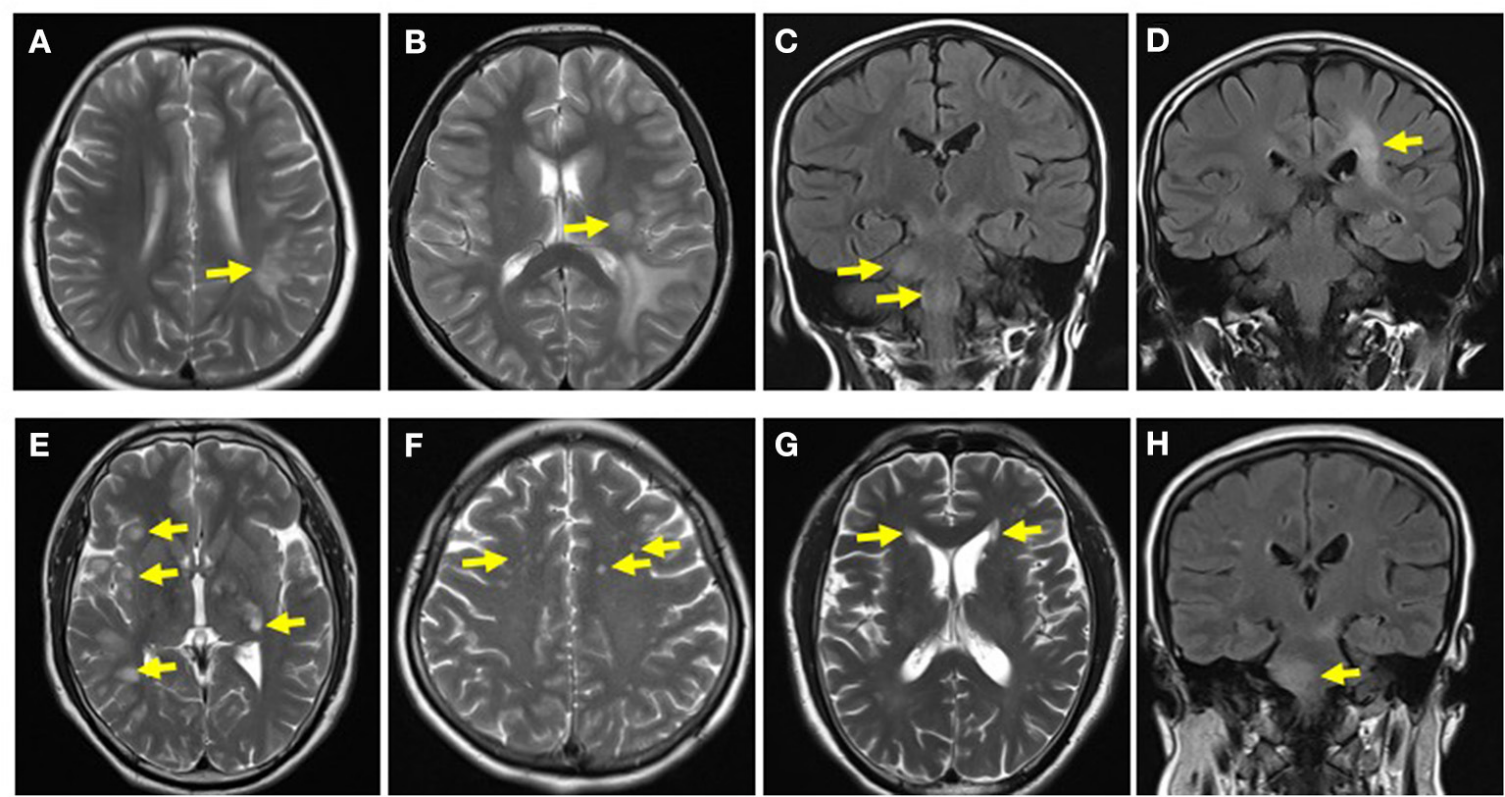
Brain MRI of pediatric and adult patients. (A) Subcortical white matter lesion in a pediatric patient. (B) Deep gray matter in a pediatric patient. (C) Infratentorial lesion in a pediatric patient. (D) Periventricular lesion in a pediatric patient. (E) Subcortical white matter and deep gray matter lesions in an adult patient. (F) Centrum semiovale lesions in an adult patient. (G) Periventricular lesions in an adult patient. (H) Infratentorial lesions in an adult patient.
